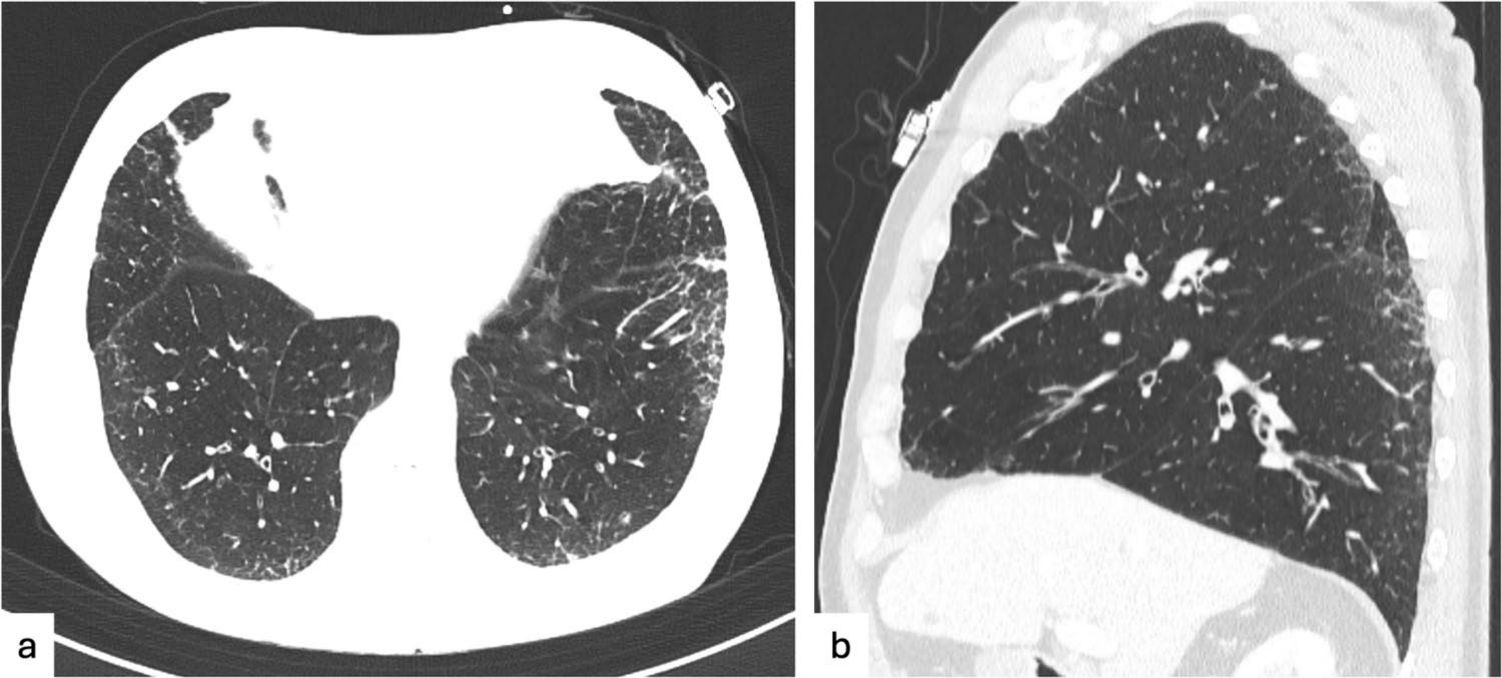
Seventy-three-year-old male patient who performed a cardiac CT scan, revealing subpleural bilateral reticulations and ground glass opacities, with a left lower lobe predominance, consistent with ILAs
